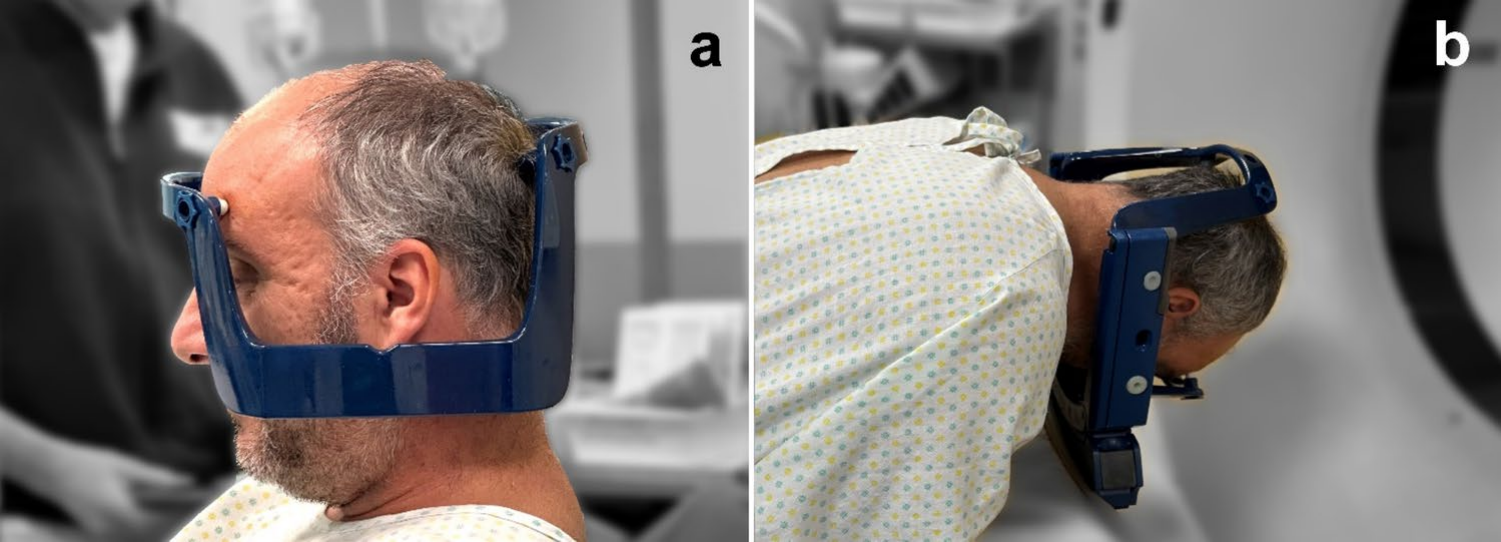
a Helmet positioning with inversion of the major axis in the anteroposterior direction. b Patient in prone position during CT scan
